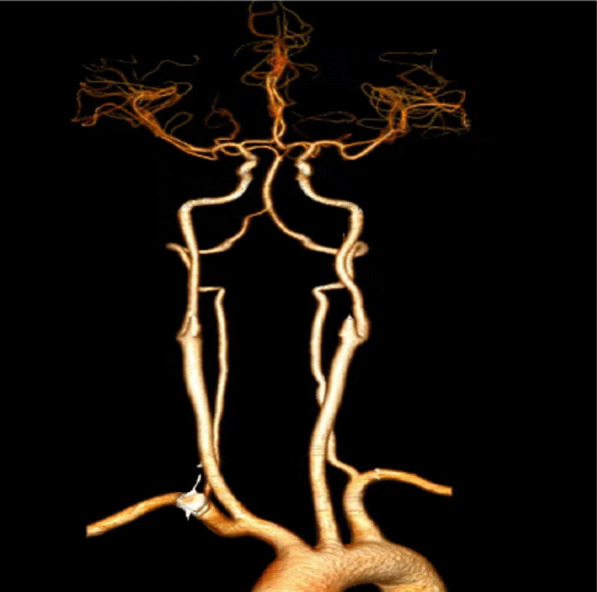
Computed tomography angiography (CTA) images showing unremarkable artery of bilateral subclavian, common carotid, internal carotid and vertebral arteries
